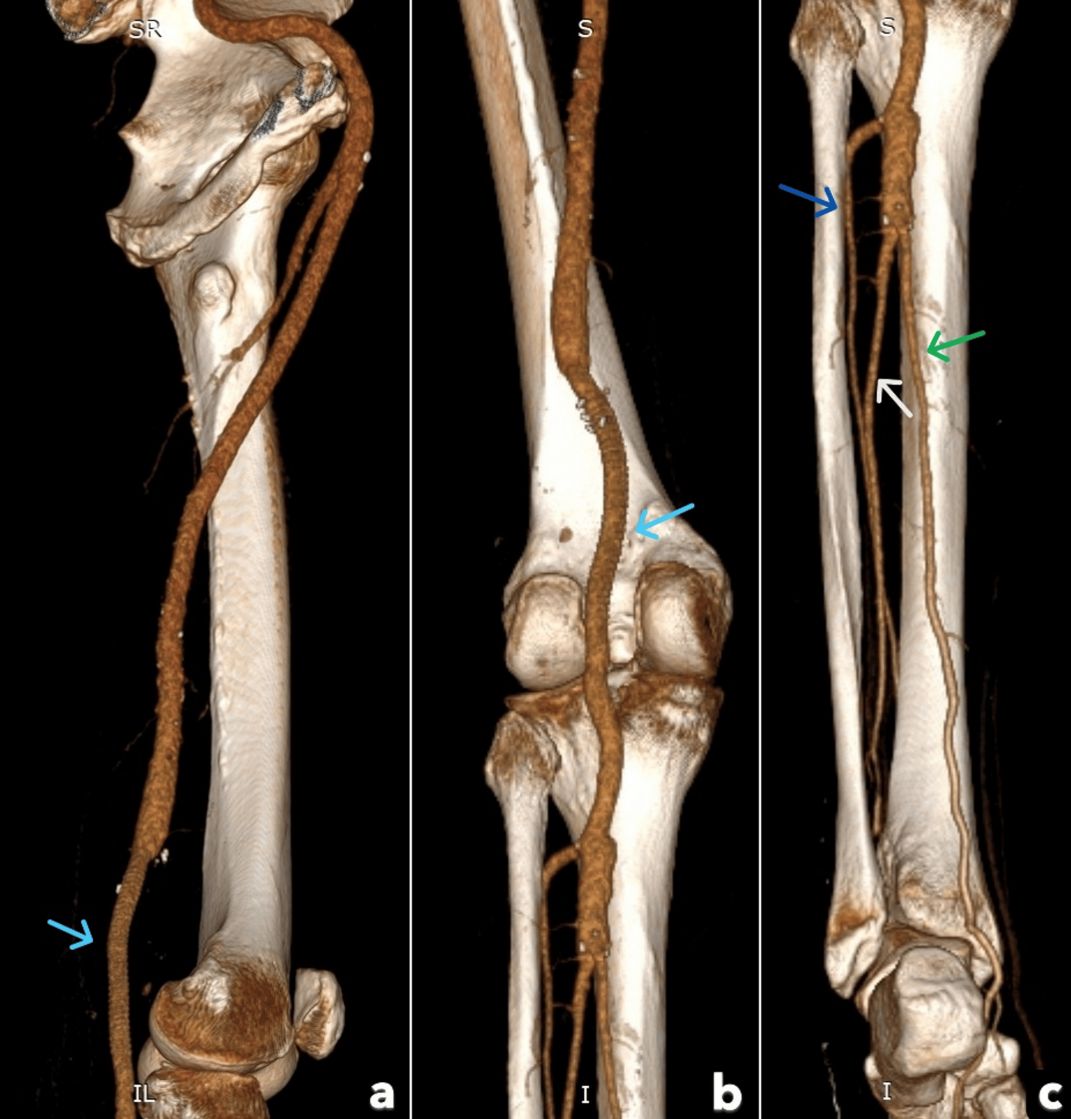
The post-operative CTA showed graft patency with restored distal vessel run-off Patent popliteal artery interposition graft (Light blue arrow); Patent anterior tibial artery (Blue arrow); Patent posterior tibial artery (Green arrow); Patent peroneal artery (White arrow).
